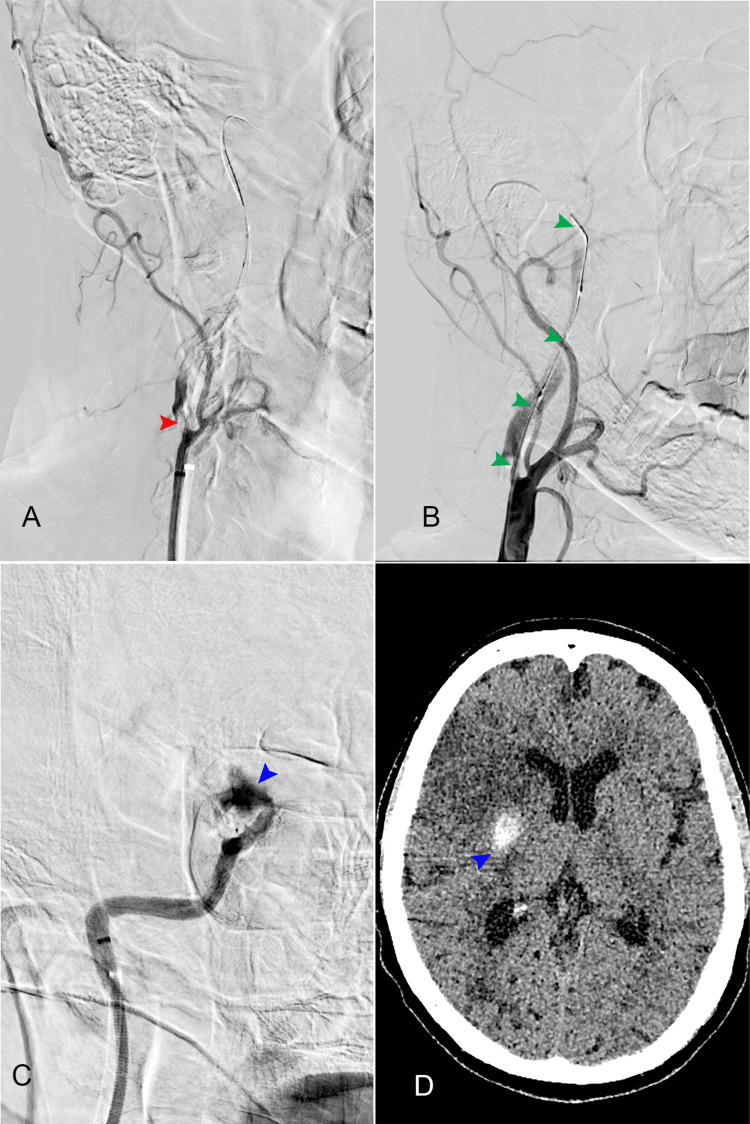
Angiography images from the thrombectomy procedure. (A) Right carotid artery bifurcation and occlusion of the internal carotid artery; red arrow: the obstruction near the bifurcation; (B) green arrows: partial visualization of the right internal carotid artery in the proximal segments; (C) blue arrow: blood extravasation during the procedure at the carotid T level; (D) CT scan performed 24 hours after the procedure, depicting intracerebral hemorrhage (blue arrow).
